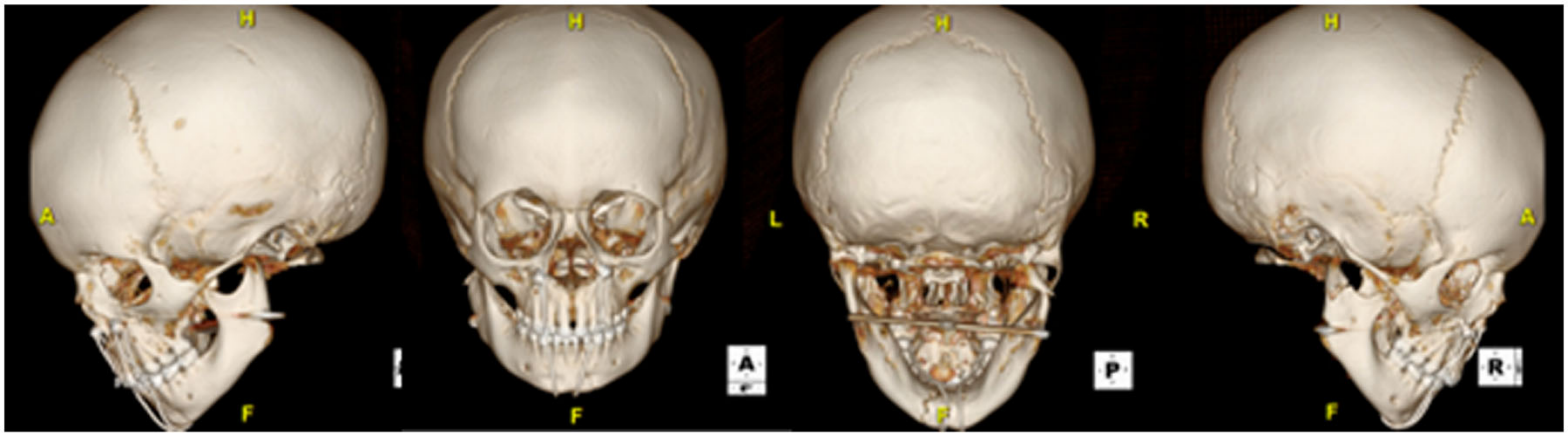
The immediate post-operative CT maxillofacial scan revealed anatomic reduction of the fracture Sites.
